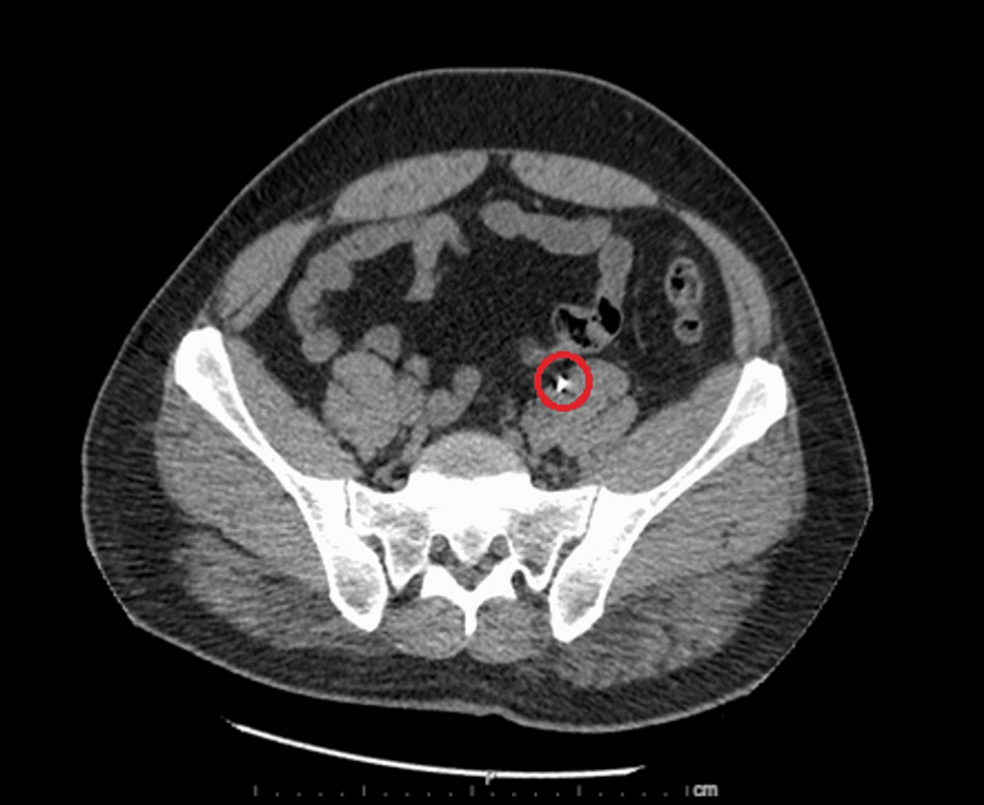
Axial view of CT abdomen pelvis without IV contrast. Red circle marking 9 mm left pelvic calcification, possible distal ureteral stone without hydronephrosis or hydroureter.
